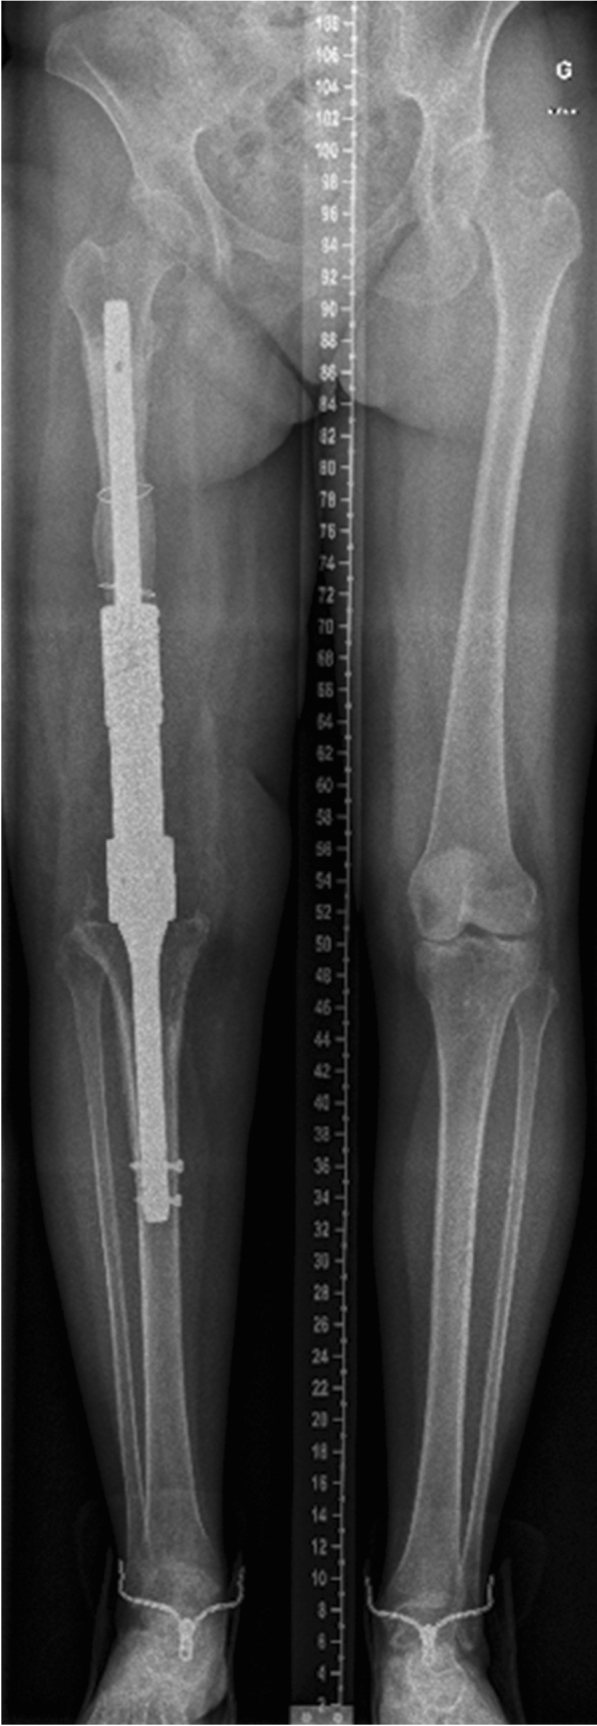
Standing whole leg radiograph of KA using OKA at 1 year
